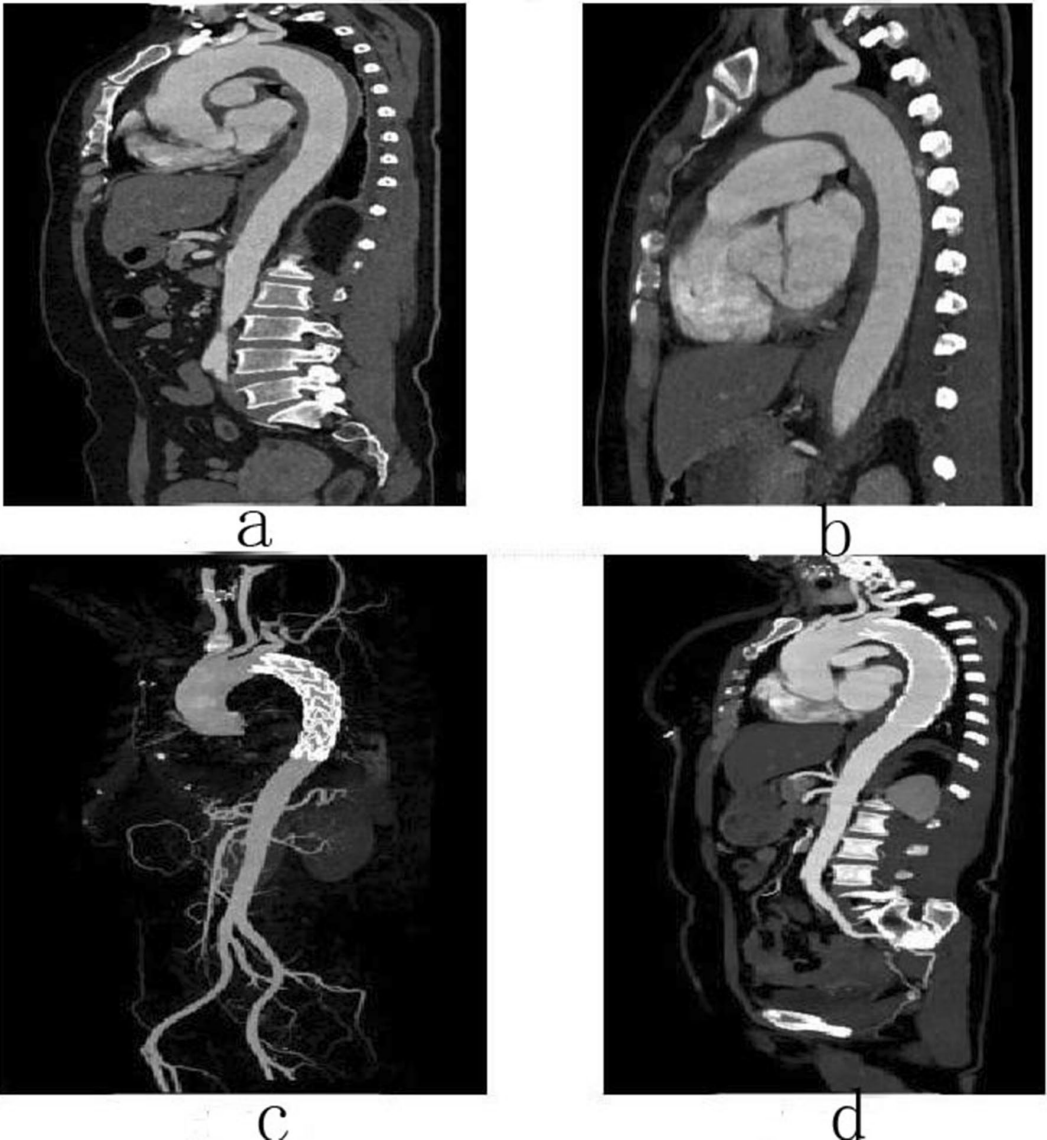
Female, 49 years old, admitted to hospital for “sudden tearing pain in low back for 48 h”, Diagnosis: Stanford type B aortic dissection; Treatment: IMH TEVAR treatment. CTA of the patient in different periods: (a) Admission; (b) 12 days after admission; (c) 2 days after surgery; (d) 4 months after surgery.
